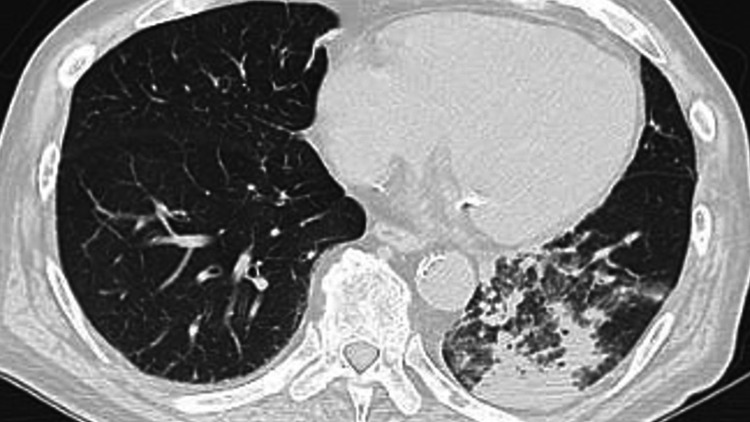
Initial chest computed tomography scan showing opacity in the left lower lung zone at admission.
